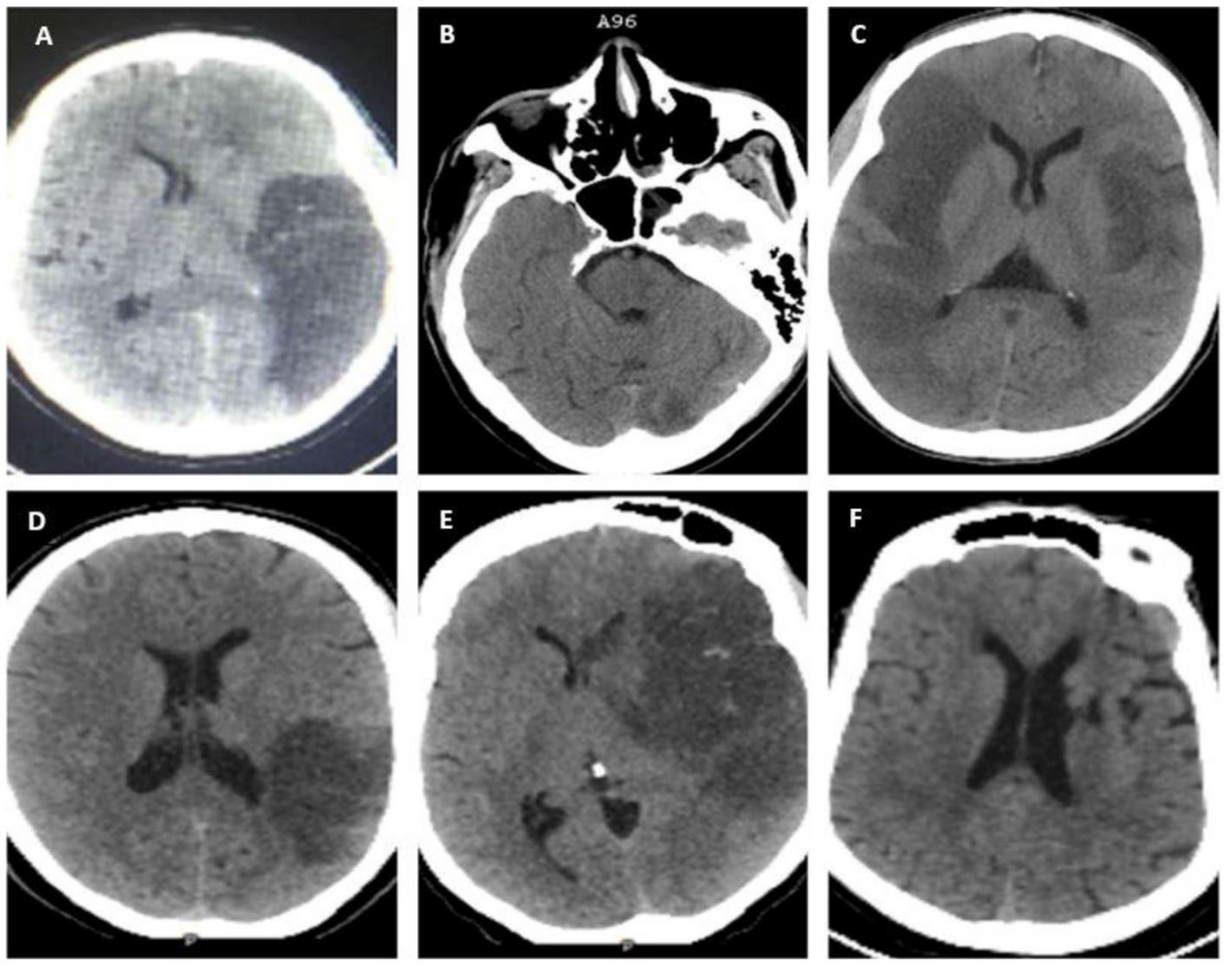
Non-contrast brain computed tomography (NCCT) scans showing evidence of ischemic infarcts in various vascular territories; (A) left distal middle cerebral artery (MCA) M1 with HI 1; (B) basilar and left posterior cerebral artery (PCA) infarction with a hyperdense basilar sign; (C) bilateral MCA: right M1 and left distal M1/ proximal M2; (D) inferior division M2 MCA with HI 1; (E) left proximal M1 with HI 1; and (F) left Lacunar infarct (non-LVO).
